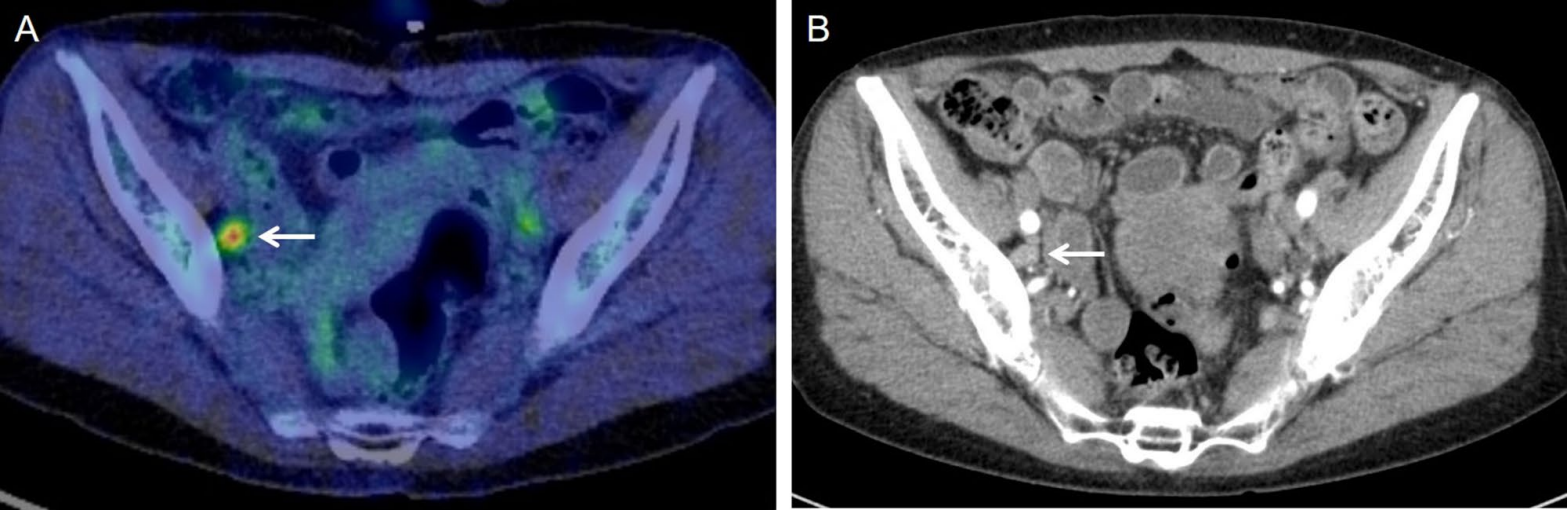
A 66-year-old woman with stage IIIC1 cervical squamous cell cancer. (A) ^18^F-FDG PET/CT image shows positive lymph nodes on ^18^F-FDG PET in a right pelvic lymph node (arrow). (B) CT image shows the lymph node (arrow) of size 0.78 cm (< 1 cm).
